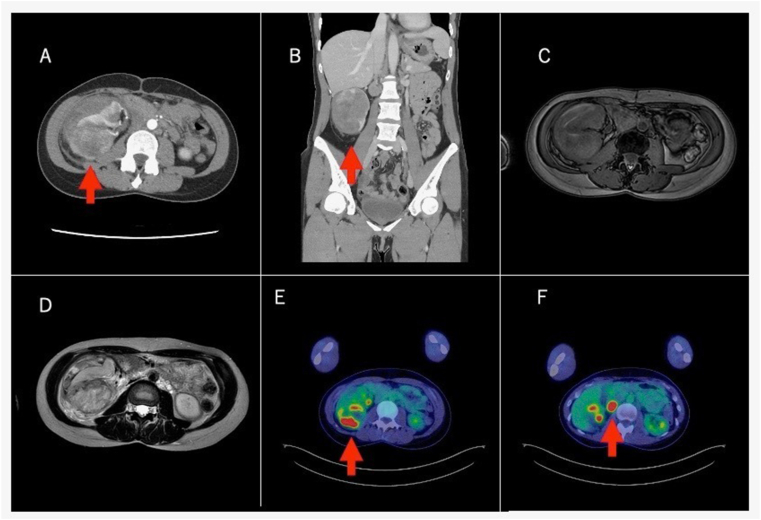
Radiologic findings. Contrast-enhanced computed tomography revealed a 6-cm right renal tumor and retroperitoneal hematoma (A, B). T1-weighted magnetic resonance imaging (C) and T2-weighted magnetic resonance imaging (D) revealing no fat tissue in the tumor. F-18 fluorodeoxyglucose positron emission tomography/computed tomography revealed strong uptake in the right renal tumor with a maximum standardized uptake value of 13.39 (E) and in the hilar lymph node with an uptake value of 15.27 (F).
